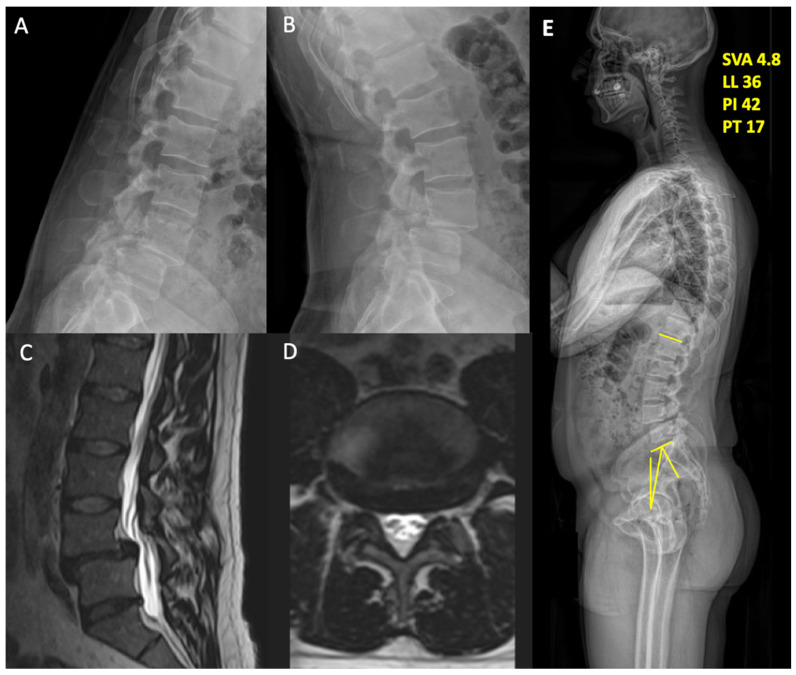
(A) Flexion and (B) extension views of standing lumbar x-rays, demonstrating the pars defect and the L4/5 grade 2 spondylolesthesis. (C) Sagittal and (D) axial cuts of T2 MRI showing the bilateral lateral recess and foraminal stenosis. (E) Lateral whole-spine film demonstrating the adequate sagittal and spinopelvic balance preoperatively.
